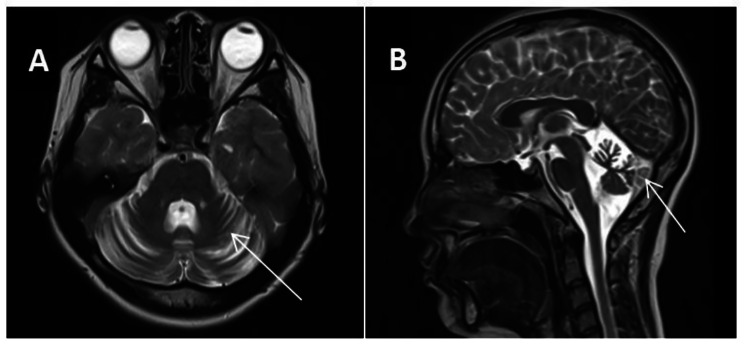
(A) Brain MRI axial sections demonstrated a considerable enlargement of the cerebellar sulcus (arrows); (B) Brain MRI sagittal sections demonstrated a clear atrophy of the cerebellum
